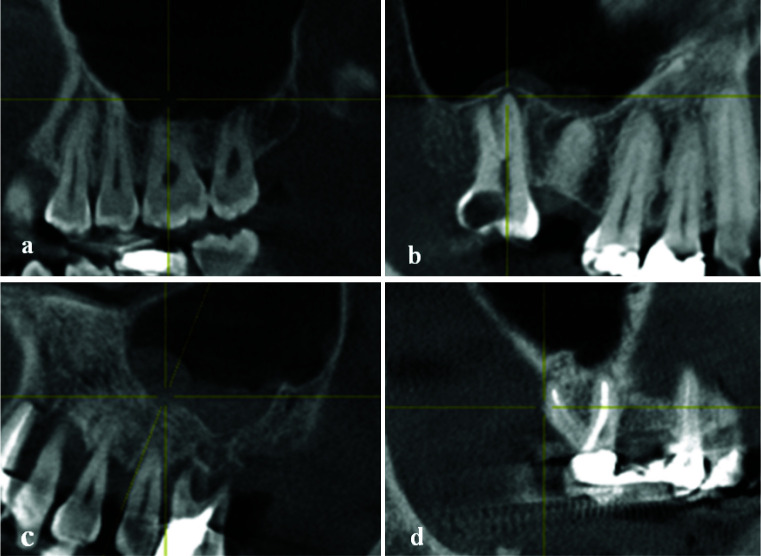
Changes in maxillary sinus observed in Cone-beam computed tomography (CBCT) imaging, a: Absence of alteration, b: Mucosal thickening, c: Sinus polyp, d: Periostitis
